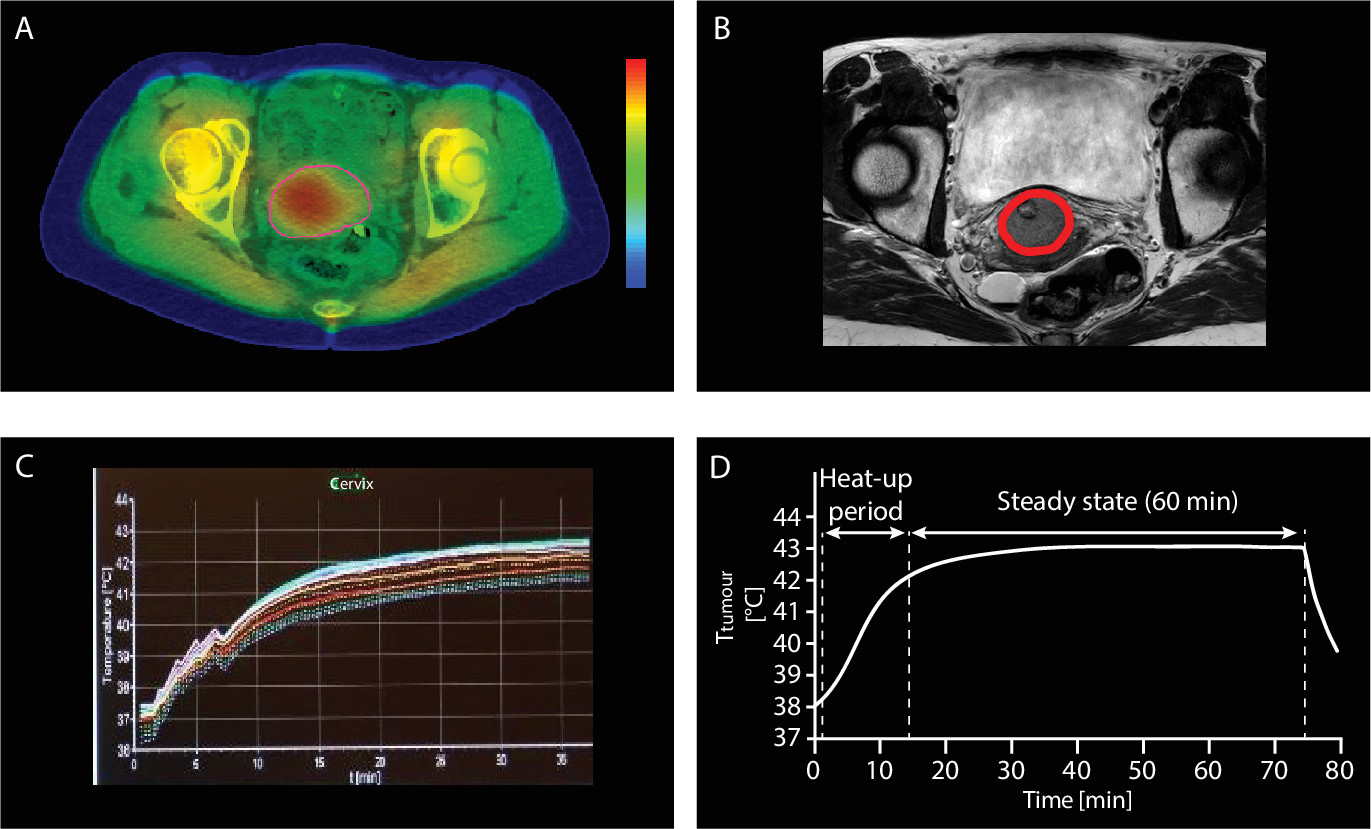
Hyperthermia treatment planning and temperature during treatment. (A) Hyperthermia treatment planning with the cervical tumor contoured in red on a dedicated hyperthermia CT scan with thermal probes in situ made directly before hyperthermia treatment. Also shown are the hot (red area) and cold (green area) spots. (B) MRI scan as help for appropriate contouring of the tumor on CT. (C) Real tumor temperature profile containing temperature readings of target area and surrounding areas during treatment. (D) Simplified tumor temperature profile during treatment.
